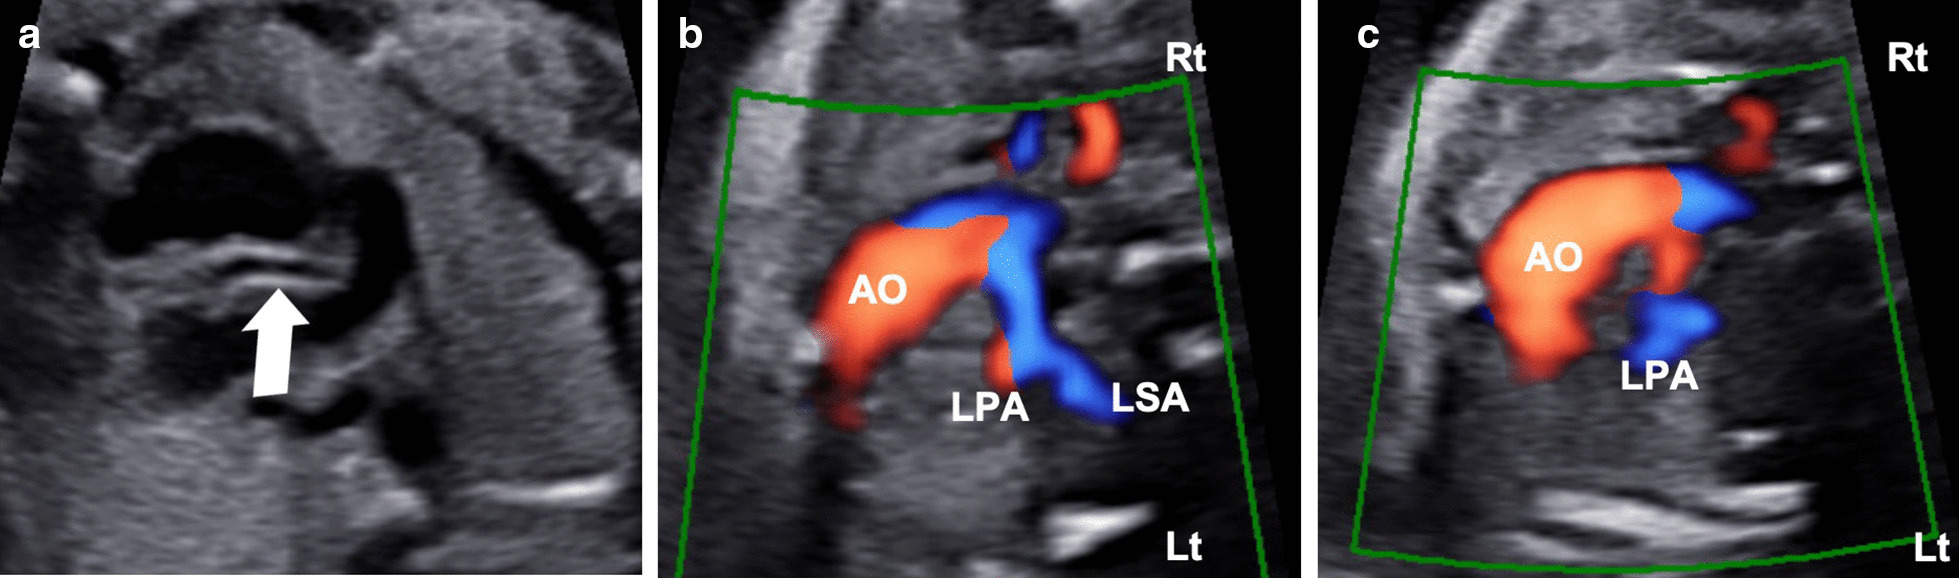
Prenatal echocardiogram showing an atretic main pulmonary artery giving rise to the right pulmonary artery (arrow) (a), left subclavian artery (LSA) from aortic arch (b), and left pulmonary artery (LPA) arising from the left subclavian artery (c)
